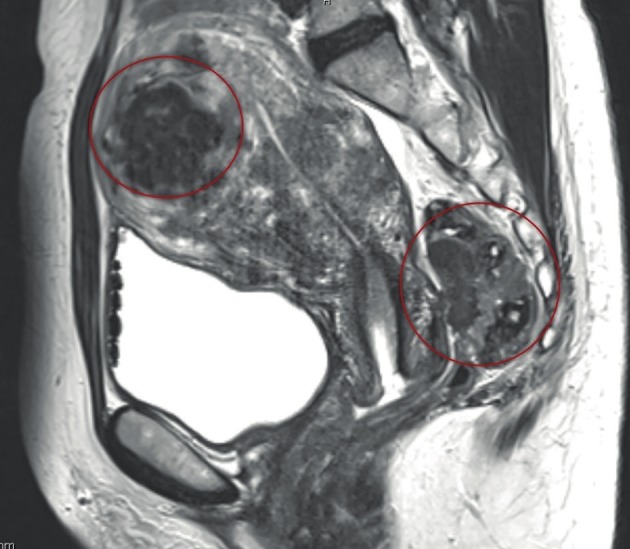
MRI picture of the pelvis. DE in the rectum with small, dense adhesions between the posterior wall of the cervix and ante- rior wall of the rectum (right circle) and adenomyosis (left circle).
